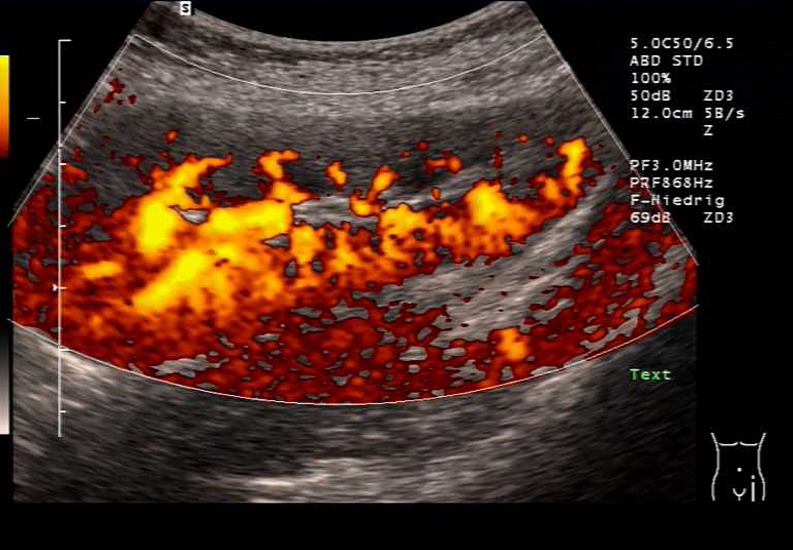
Longitudinal power Doppler ultrasonogram of a patient with transplant pyelonephritis showing vascular hypoperfusion in the cortex
